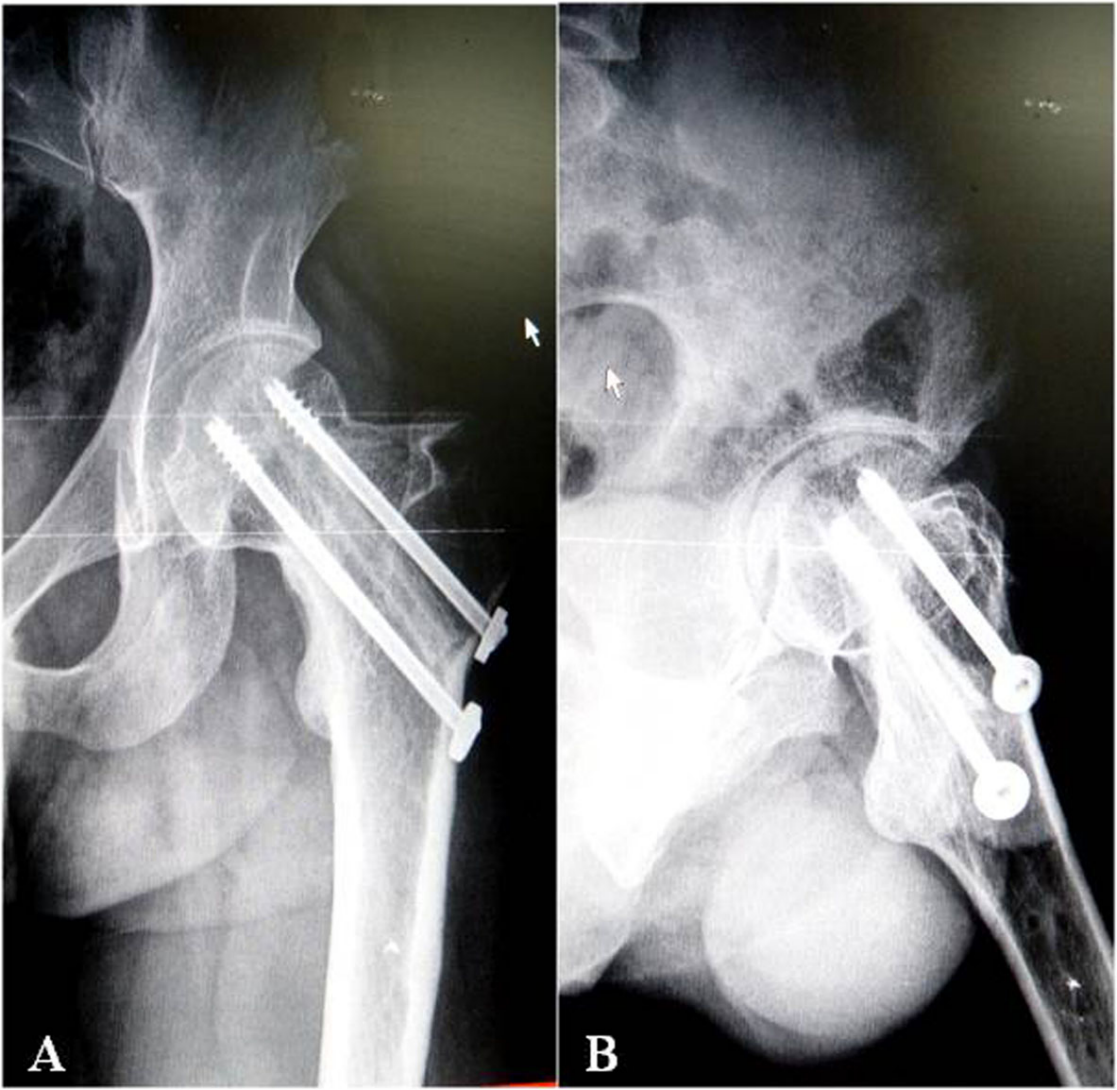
Six months after autologous iliac bone graft plus cannulated screw fixation, left hip joint reduction, cannulated nail fixation, blurred fracture line, blurred humeral bone, and good fracture healing. a hip X-ray anterior piece, b hip joint X-ray lateral slice
